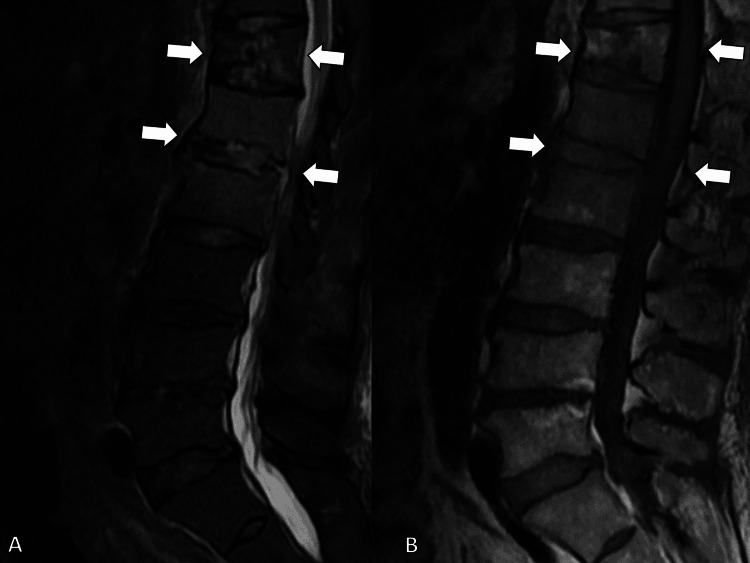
Magnetic resonance imaging of the lumbar spine revealing signal changes at the L1/L2 level (white arrows). (A) Short τ inversion recovery image, and (B) T1-attenuated image.
